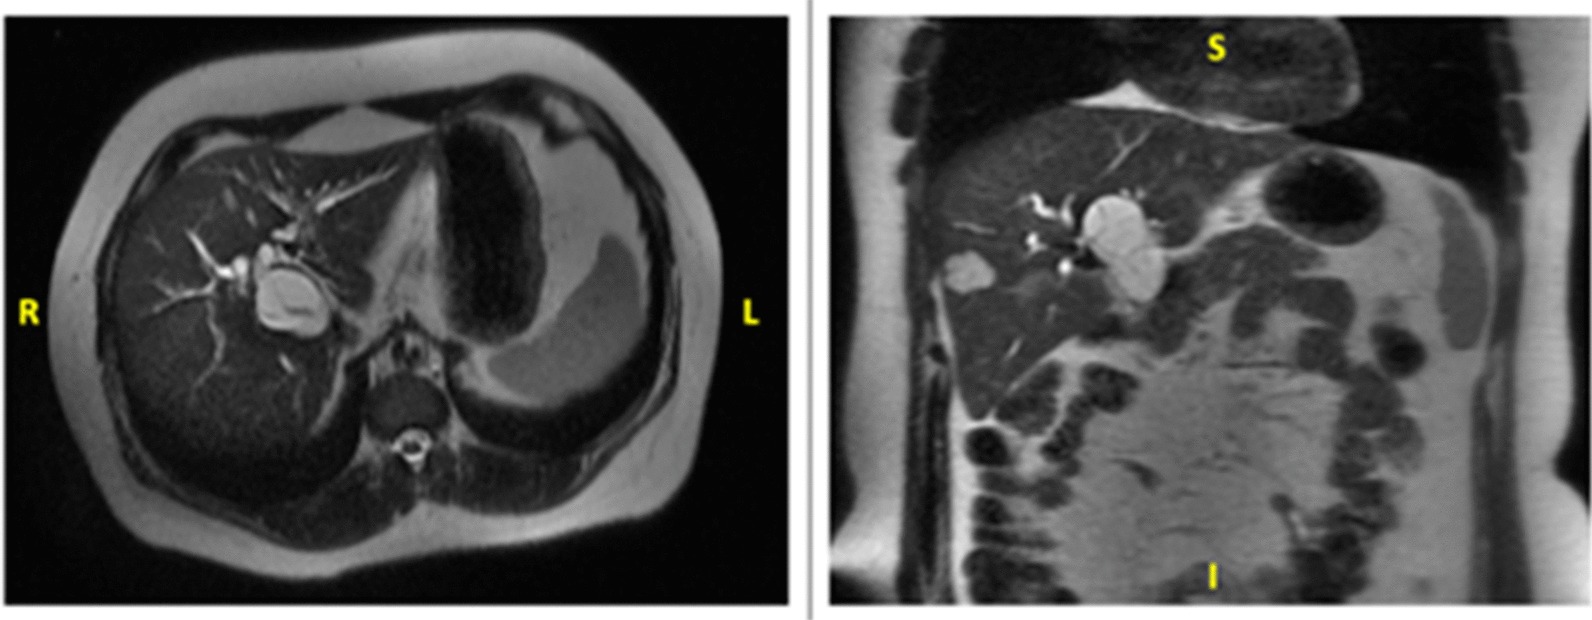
Abdominal MRI showing a hypodense lesion in segment V/VIII of the liver with few internal septations (axial and coronal planes)
